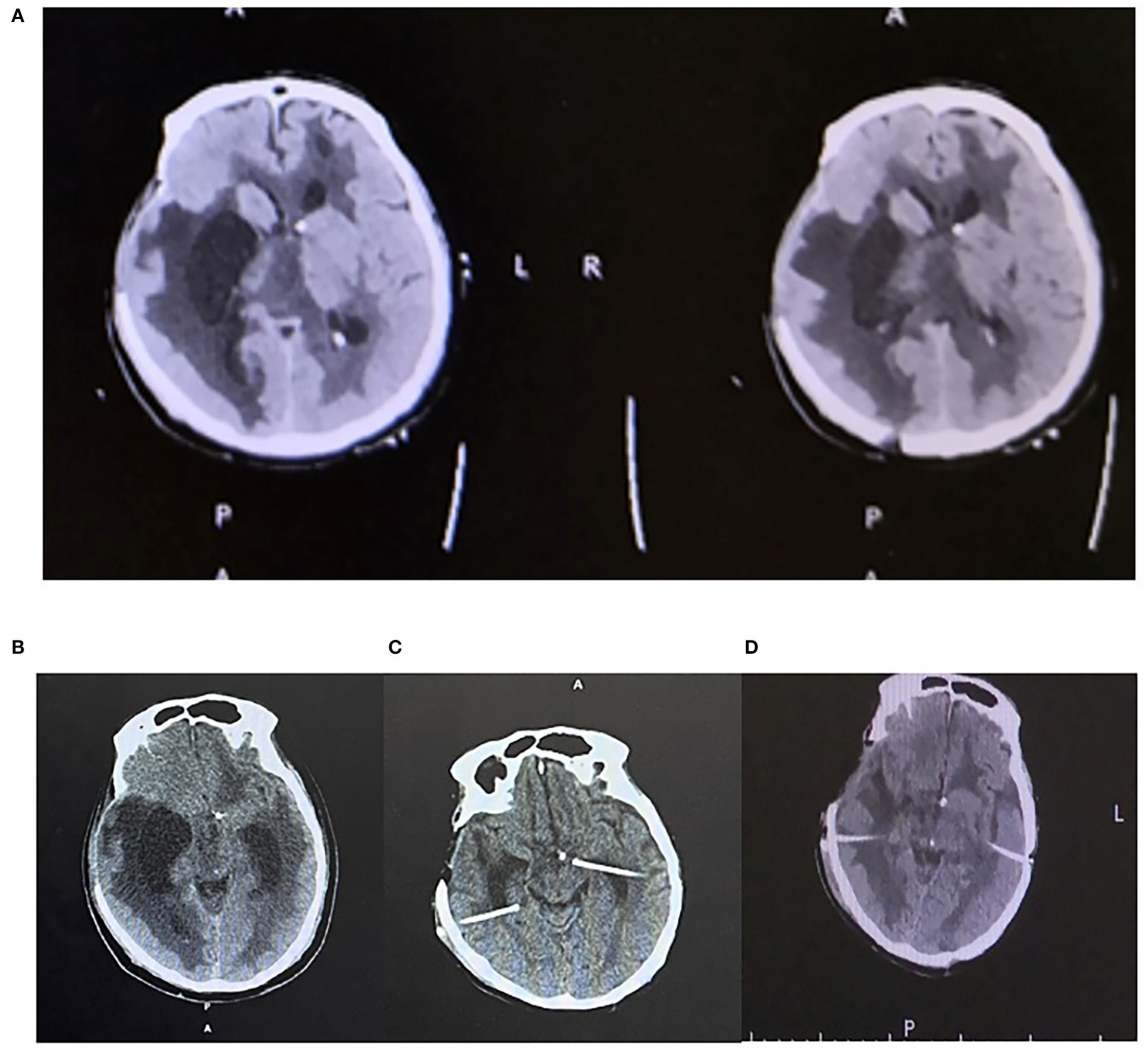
(A) Axial plane CT head showing multiple loculations in the ventricle. (B) Axial CT head showing enlarged ventricles prior to B/L shunt. (C) Axial head CT showing ommaya pump placement on B/L temporal horns. (D) Axial head CT after B/L VP shunt creation.
